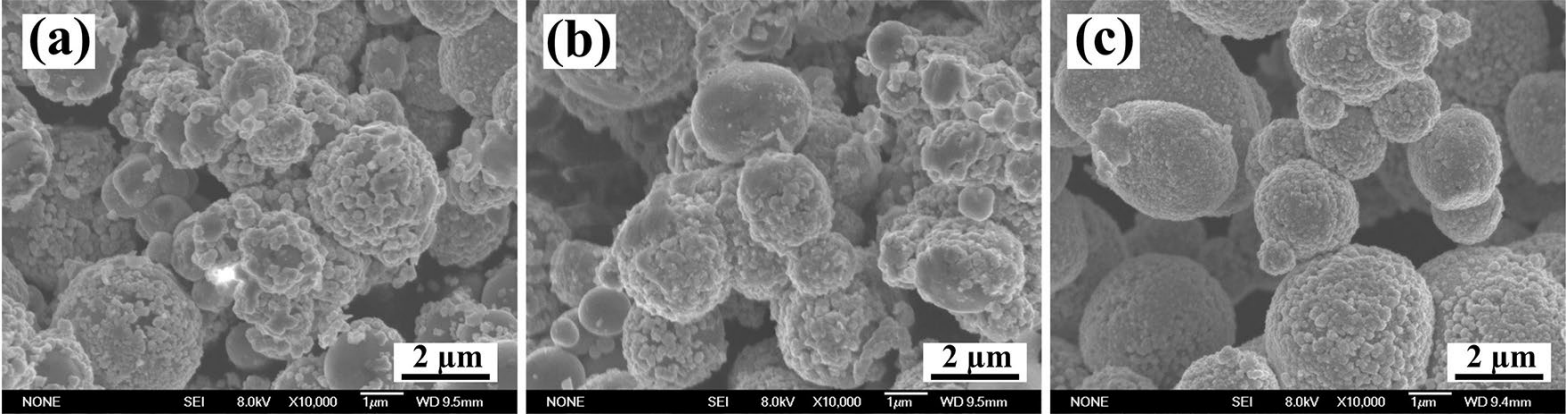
Morphologies of plating coating TiC_p_ with various stirring method (a) manual stirring (b) ultrasonic stirring (c) magnetic stirring.
